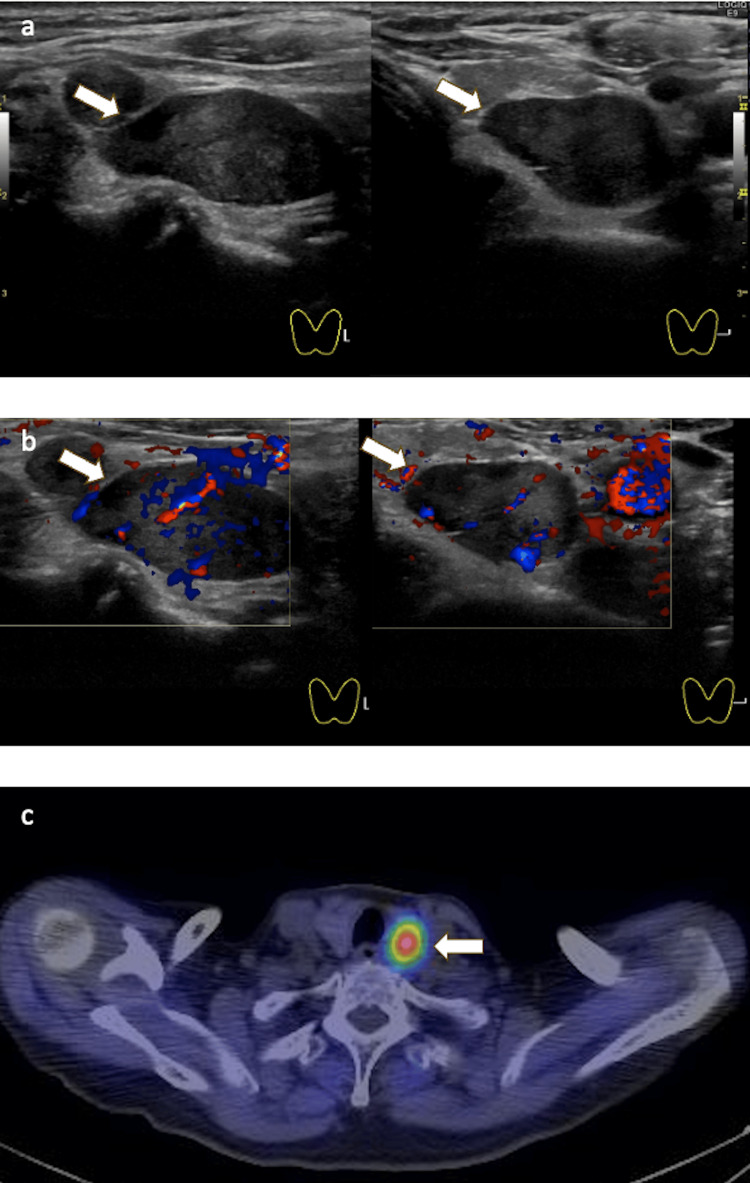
Parathyroid lesion. (a) A neck ultrasound revealed a well-demarcated hypoechoic mass measuring 26×21×12 mm lateral to the left thyroid lobe, with slightly heterogeneous internal echotexture. (b) Color Doppler ultrasound showed rich vascularity within the lesion. (c) MIBI scintigraphy demonstrated focal radiotracer uptake in the corresponding area. Arrows indicate the tumor and MIBI-avid region.
